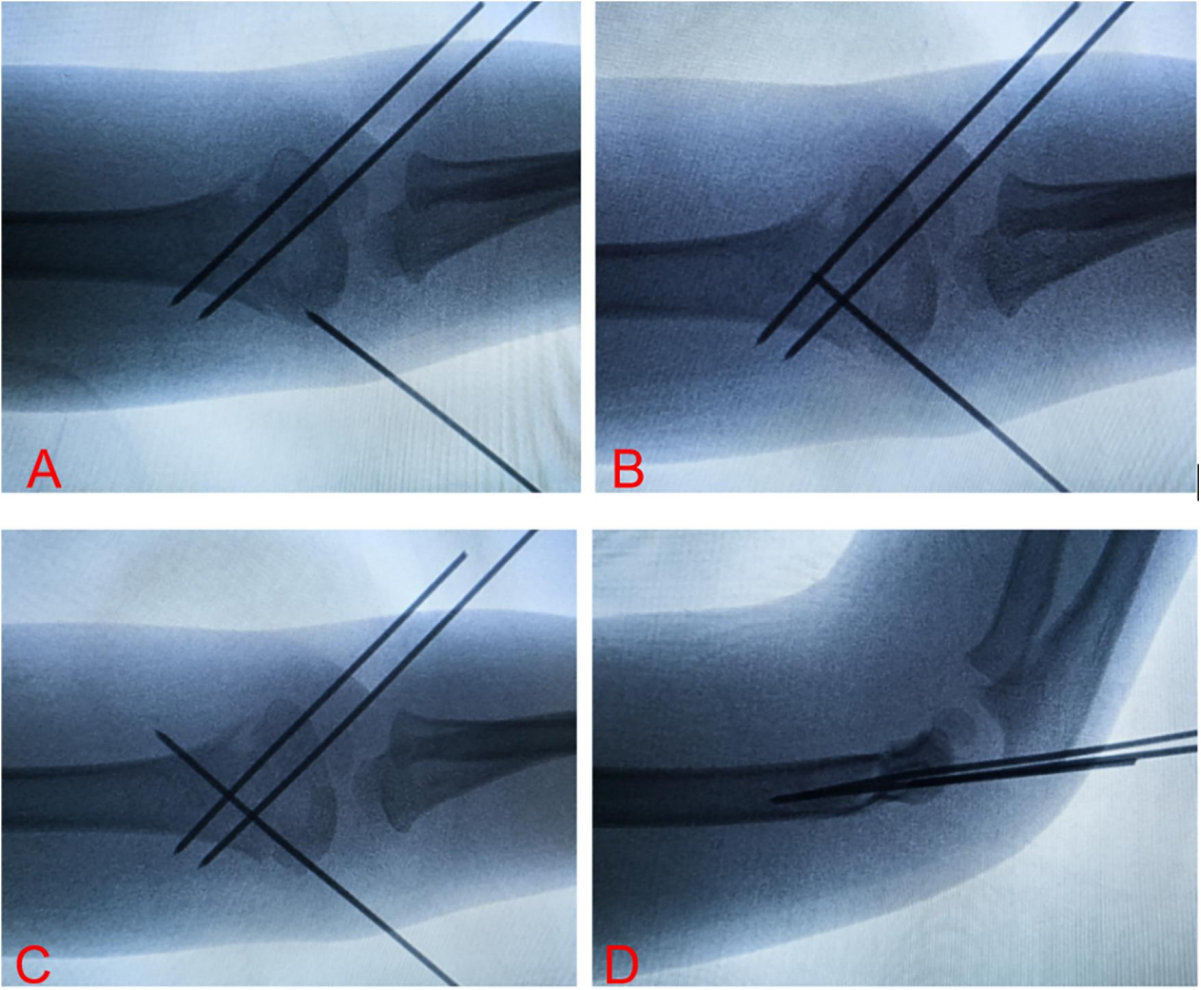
This figure shows the K-hammer surgical procedure under the guidance of a C-arm. (A,B) Show the process of slow Kirscher wire insertion at the medical condyle of the humerus, and (C,D) show the anteroposterior and lateral x-rays of the elbow joint after the completion of Kirschner wire insertion.
